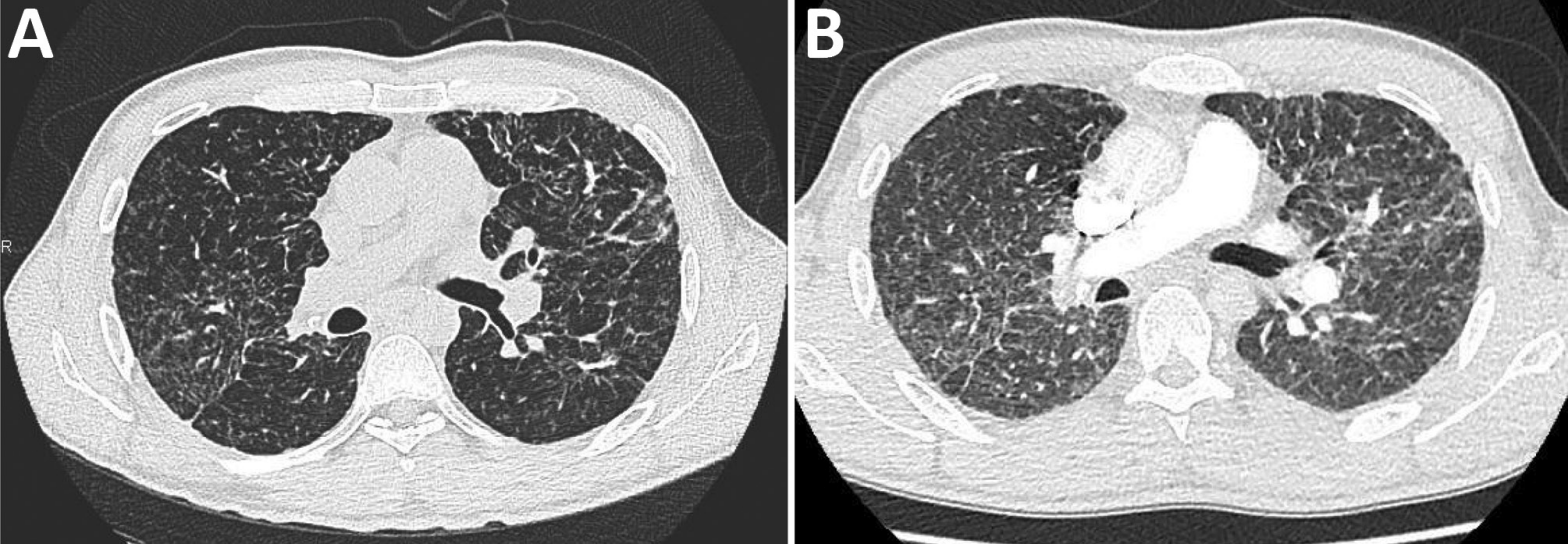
Computed tomography (CT) scans of a coronavirus disease (COVID-19) patient with sarcoidosis who had been receiving long-term hydroxychloroquine treatment, France. A) Thoracic CT scan from November 2019, showing baseline pulmonary sarcoidosis lesions. B) Thoracic CT scan performed April 4, 2020, showing diffuse ground-glass opacities characteristic of COVID-19.
